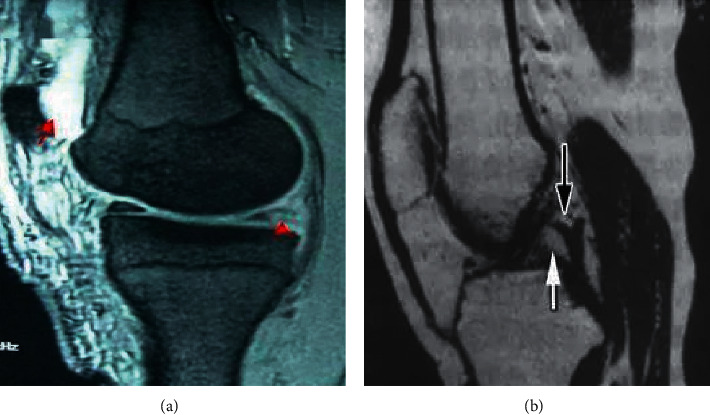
MRI T2WI images of the patients (male, 61 years old). (a) Tear of posterior corner of medial meniscus of knee joint (the arrow was the tear site); (b) MRI T2WI plain scan of posterior cruciate ligament (the arrow indicated a partial rupture of the ligament).
